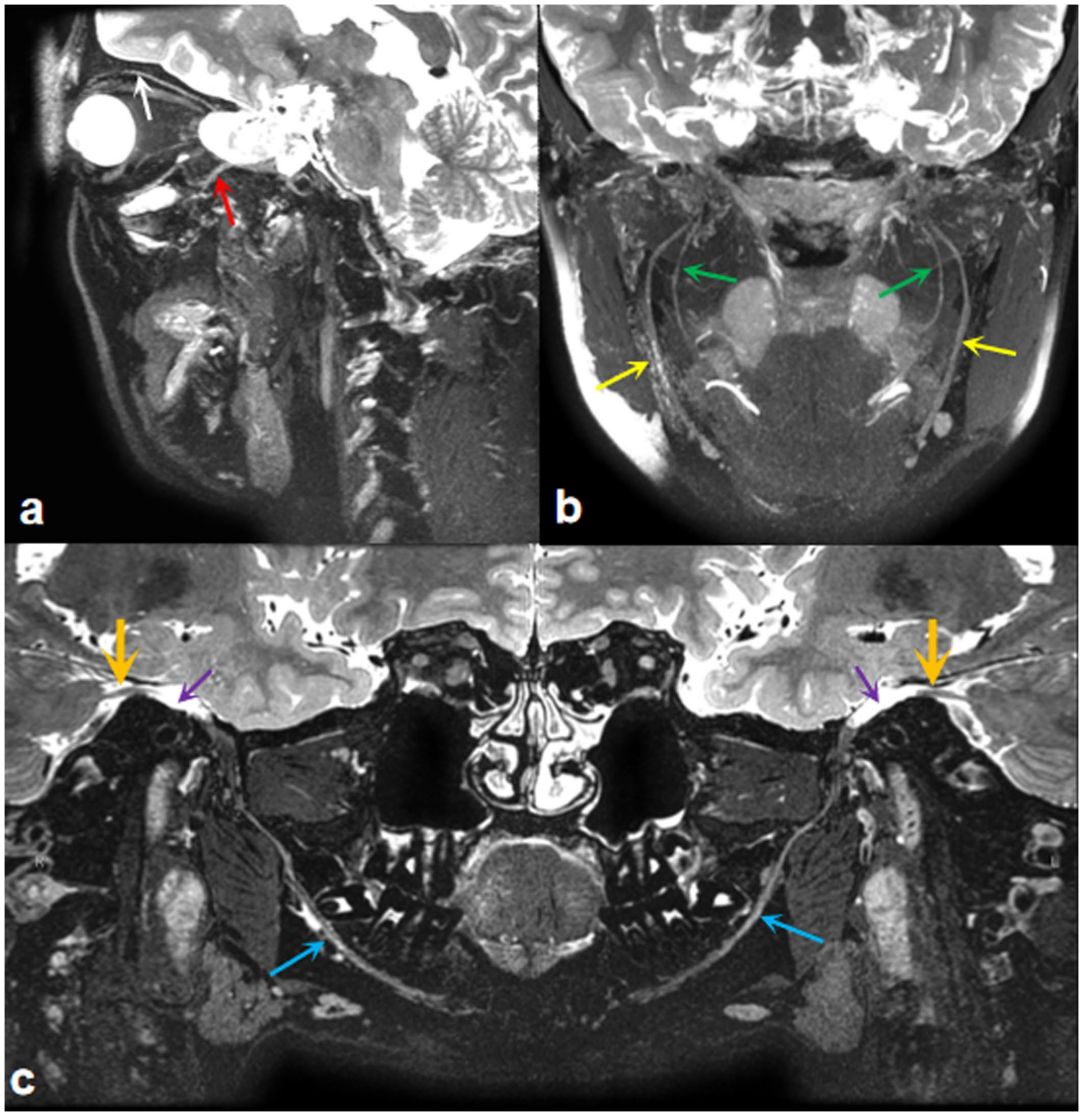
MRI imaging of the trigeminal nerve. (A) A three-dimensional T2WI-CUBE fs reconstruction image displays the ocular nerve (white arrow) and maxillary nerve (red arrow). (B) A three-dimensional T2WI-CUBE fs reconstruction image shows the branches of the mandibular nerve, inferior alveolar nerve (green arrows), and lingual nerve (yellow arrows). (C) A three-dimensional T2WI-CUBE fs reconstruction image provides a comprehensive view of the mandibular branch of the trigeminal nerve, including the trigeminal nerve itself (orange arrows), Meckel cavity (purple arrows), and mandibular nerve (blue arrows).
